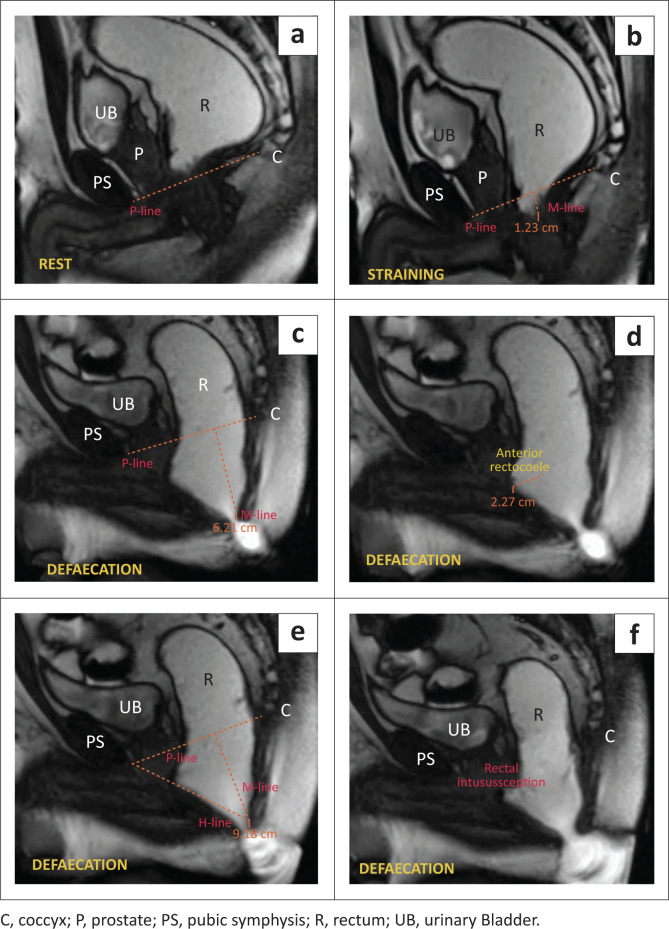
(a–e) MRI defaecogram: Rectal intussusception. (a) At rest showed no abnormality. (b) On straining showed anorectal descent with the anorectal junction 1.2 cm below the P-line. (c) The defaecation phase revealed the anorectal junction 6.2 cm below the P-line, representing anorectal descent. (d) Defaecation phase reveals a moderate anterior rectocoele (2.27 cm). (e) The defaecation phase shows an increased H-line (9.18 cm), depicting levator hiatal widening. (f) The defaecating sequence also shows recto-rectal intussusception.
